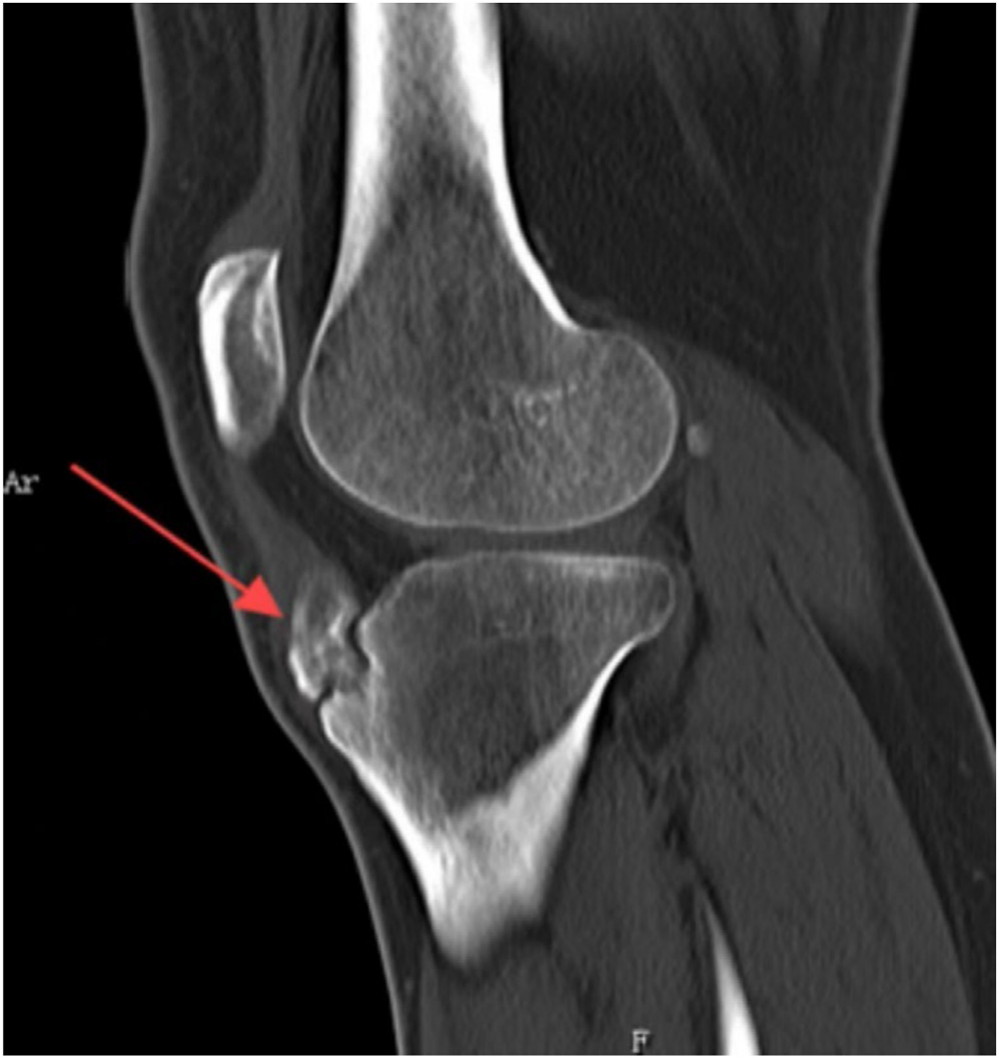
The sagittal view of the computed tomography scan of the patient’s left knee. The red arrow points to the tibial tubercle osteophyte, consistent with the characteristics of Osgood-Schlatter disease.
